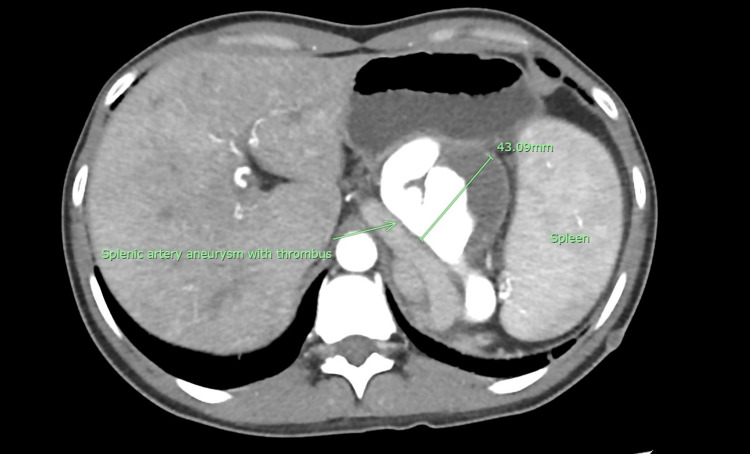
Computed tomography (CT) angiogram (axial view) showing splenic artery aneurysm (SAA) with thrombus before embolisation
